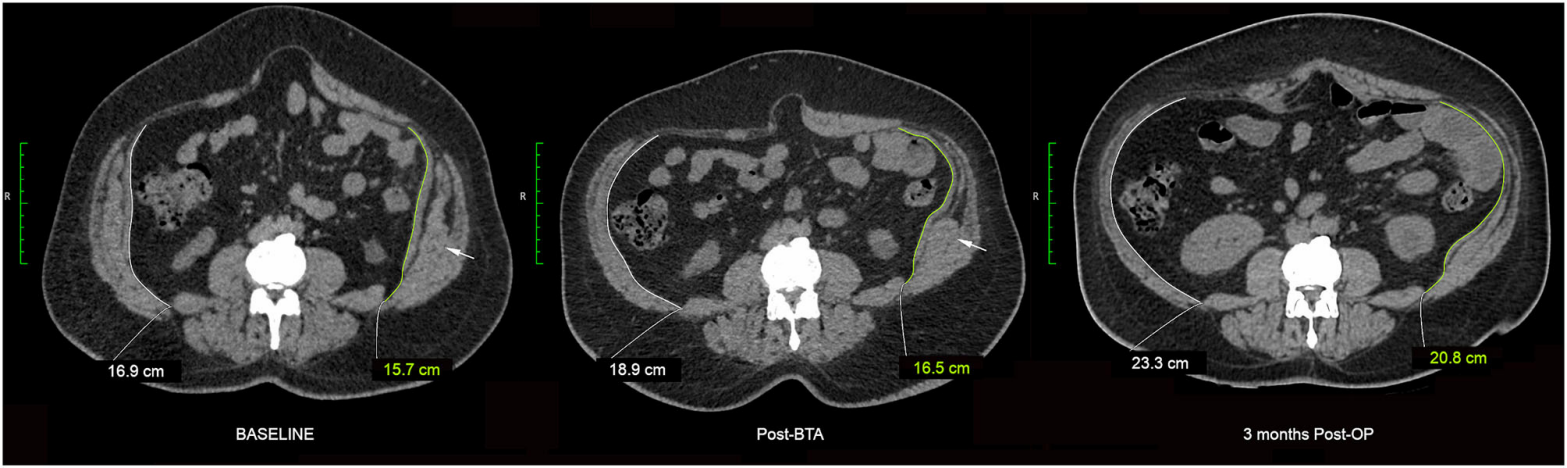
Variable BTA effect. Crunching phase images obtained at the same vertebral level are shown from the baseline diagnostic, pre-operative post-BTA and 3 months post-operative CT examinations of a patient with complex ventral hernia. Component relaxation was performed using a total of 300U BTA divided equally prior to the individual infiltration of each muscle layer of the abdominal wall in the anterior axillary line as described by Farooque et al. (1). The pre-operative post-BTA image shows variable chemical relaxation effect in the very same muscle: there is profound relaxation of the anterior segment but apparent non-relaxation of the posterior segment (white arrows) of left IO muscle. However the 3 months post-operative image subsequently shows (i) relaxation of the previously unresponsive posterior segment of left IO muscle, and (ii) an apparent increase in overall BTA effect as evidenced by a reduction in thickness and increase in axial length of the EO + IO + TA muscle complexes on both sides.
